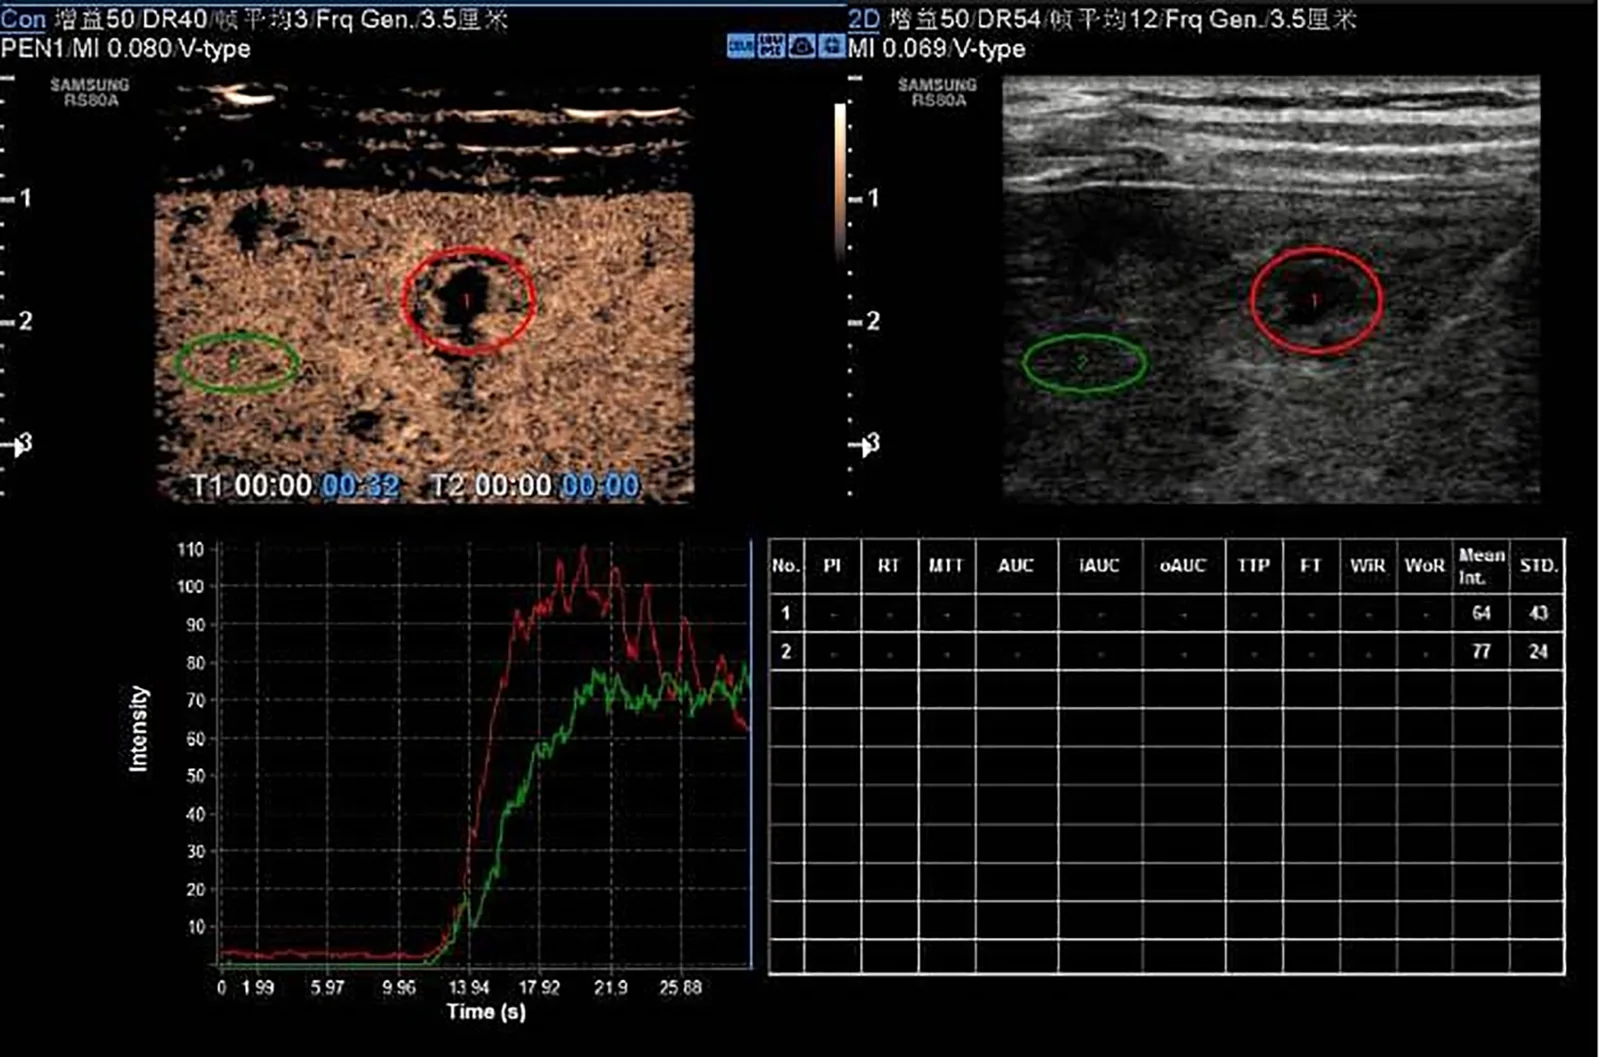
Hepatic contrast-enhanced ultrasound and quantitative analysis images. Quantitative parameters include area under the curve (AUC), inflow area under the curve (iAUC), outflow area under the curve (oAUC), time to peak (TTP), wash‑in rate (WiR), and wash‑out rate (WoR).
